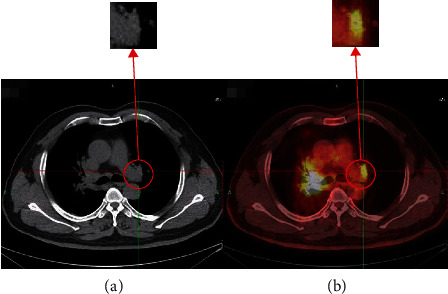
(a) Lung CT image. (b) Lung PET/CT image.
